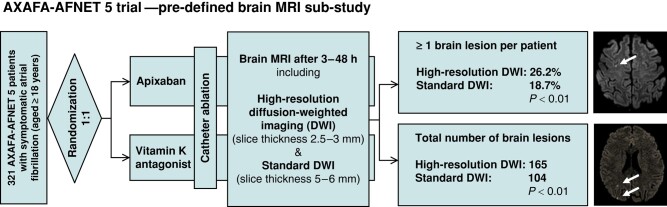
Design of the pre-defined brain MRI sub-study of the AXAFA-AFNET 5 trial also demonstrating the main results comparing MRI-detected acute ischaemic brain lesions using hr vs. standard DWI. DWI, diffusion-weighted imaging; MRI, magnetic resonance imaging.
